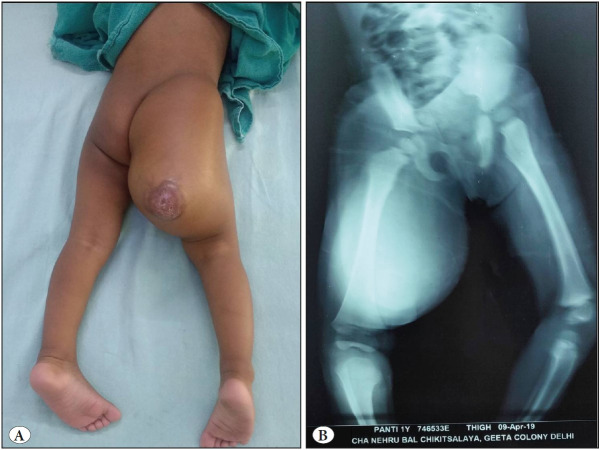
A) Clinical picture of the right gluteal and thigh mass with an area of skin ulceration. B) Radiograph shows a large radiolucent mass. Underlying bone is not involved.
